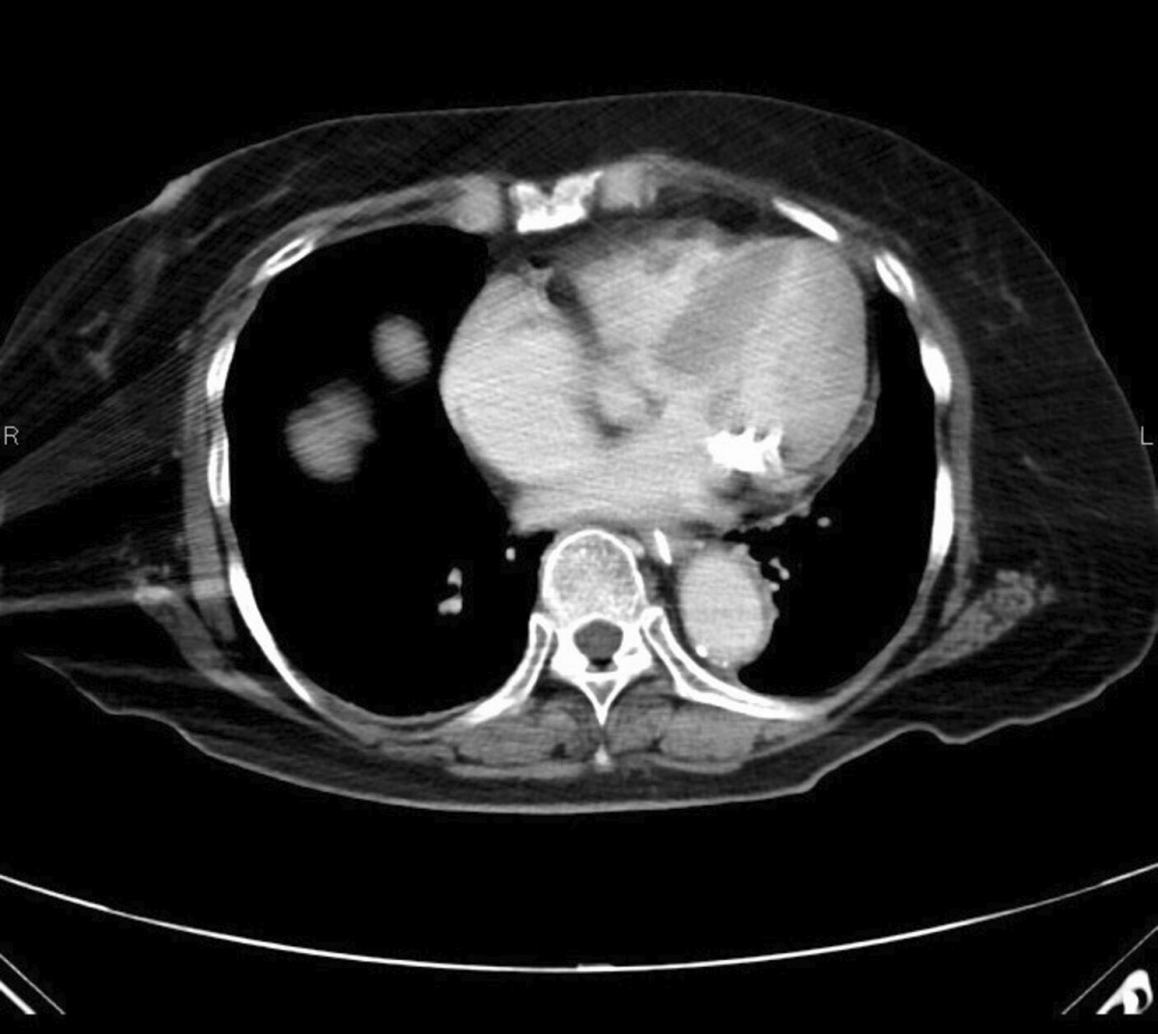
Chest computed tomography shows massive calcification of posterolateral aspect of mitral annulus.
